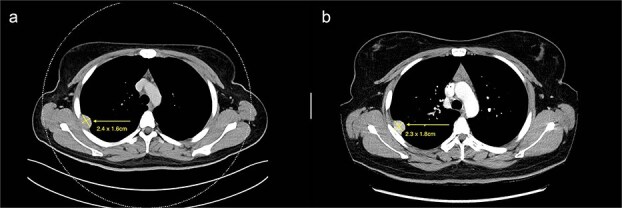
(a) Axial cut of CT thorax taken in April 2024, depicting a right sided hyperdense peripheral pleural lesion measuring 2.4 × 1.6 cm. (b) Axial cut of a repeat CT thorax taken in February 2025, showing the same hyperdense pleural lesion relatively stable in size, now measuring 2.3 × 1.8 cm.
